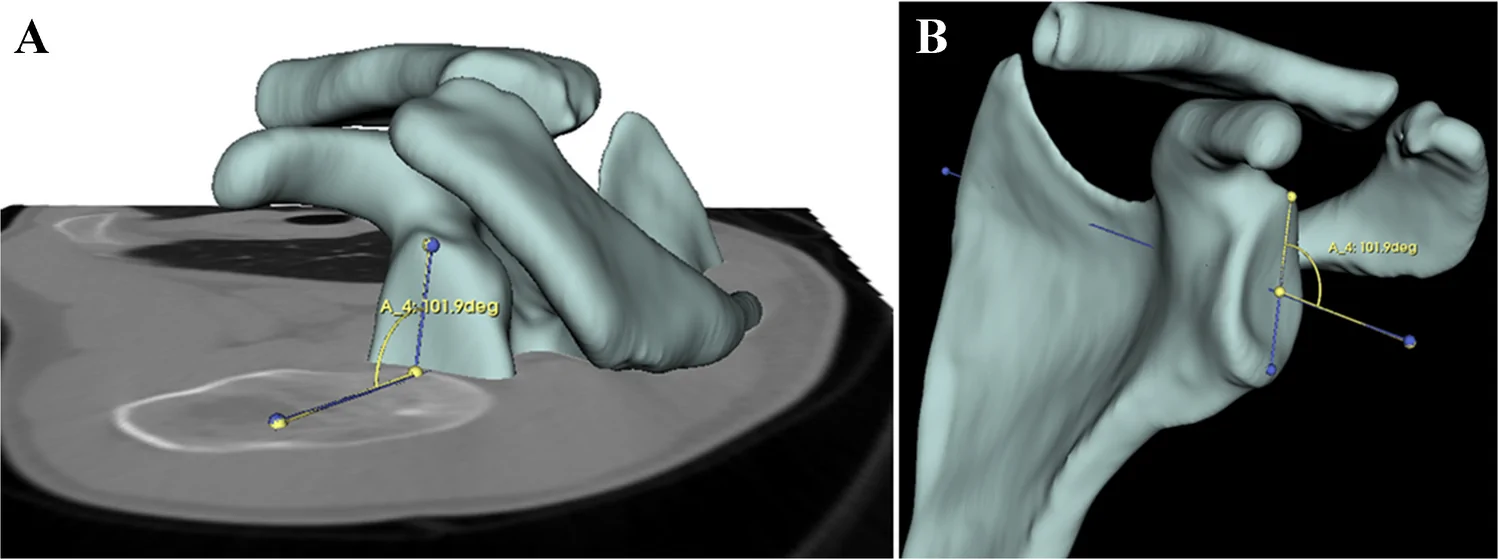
Demonstration of glenoid inclination (GI) measurement on 3D-CT. A View from lateral. B View from anterior
